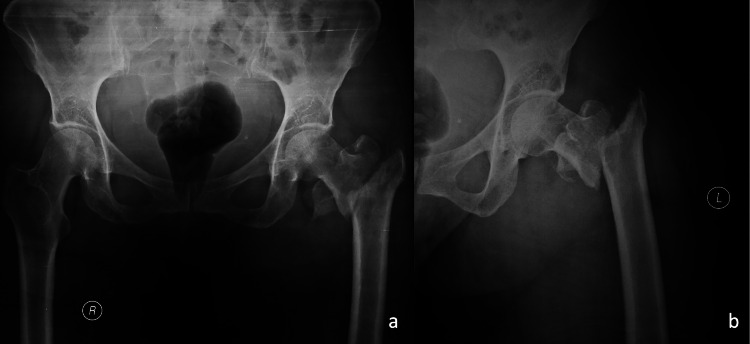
Anteroposterior pelvis (a) and lateral left hip (b) x-rays.
